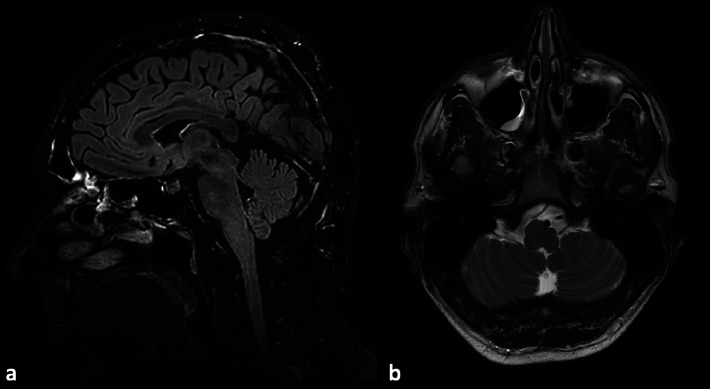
Sagittal FLAIR (a) and axial T2-weighted follow-up images (b) showing complete resolution of the vasogenic edema in the brainstem and cervical spinal cord without atrophy or residual changes.
